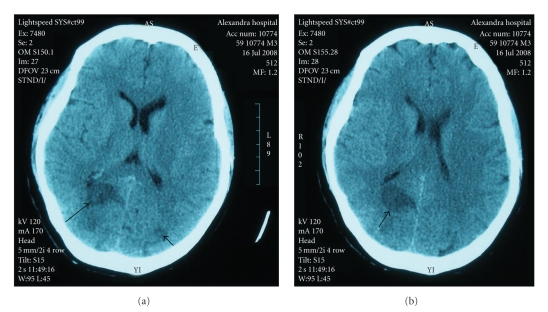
Computed tomography of the brain without contrast, obtained 24 hours after admission of the patient to the hospital: infarcts are present in both occipital lobes (arrows).
